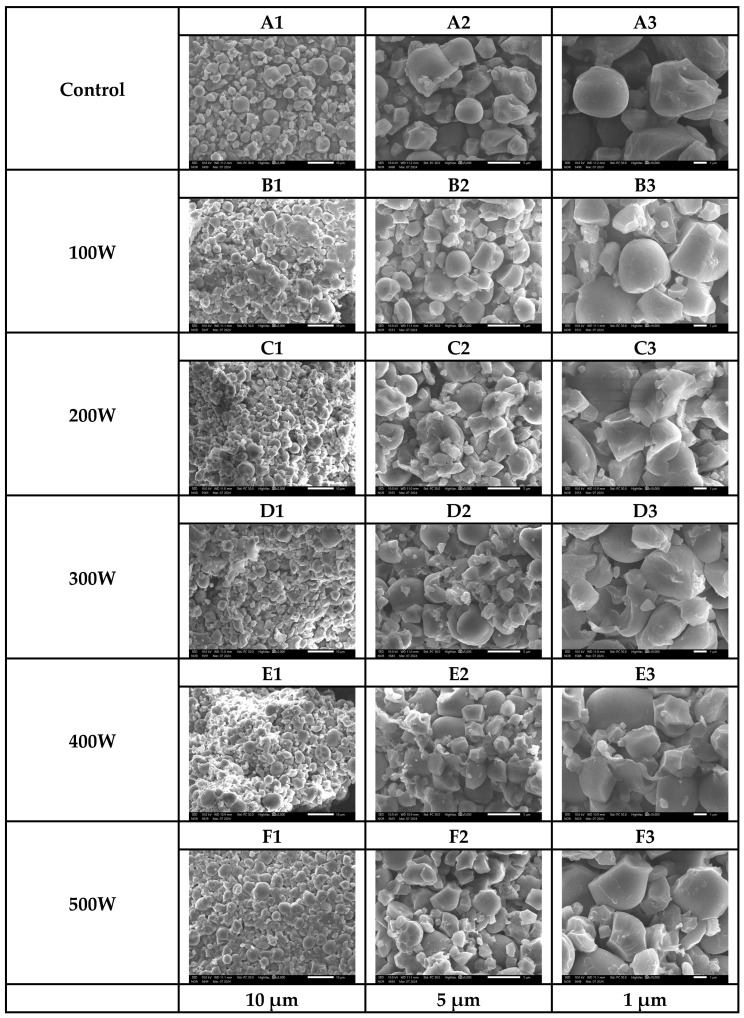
SEM images of the control (A1–A3) and ultrasound-treated samples at 100 W (B1–B3), 200 W (C1–C3), 300 W (D1–D3), 400 W (E1–E3), and 500 W (F1–F3).
